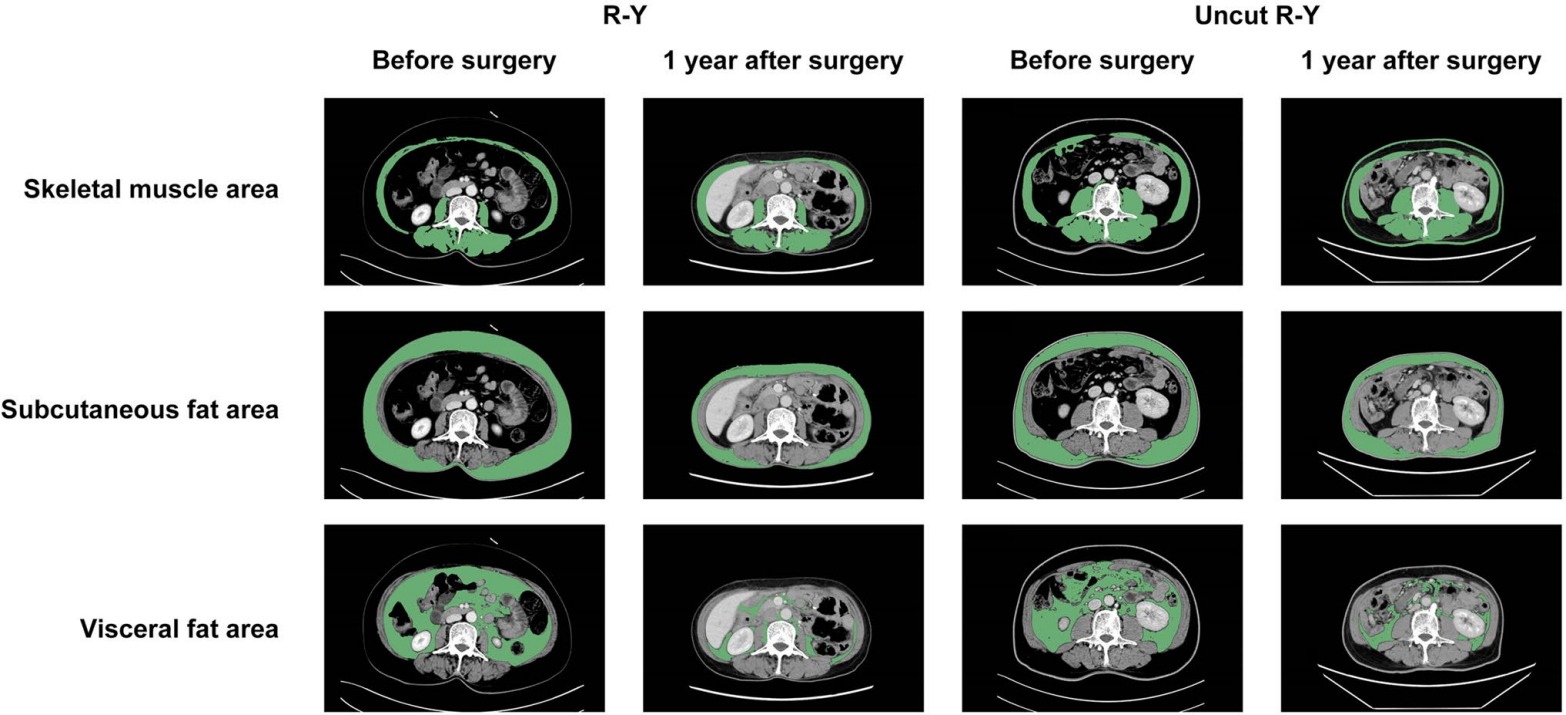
Representative image of skeletal muscle area, subcutaneous fat area, and visceral fat area from CT-based analyses at the level of the fourth lumbar vertebra (L4)
